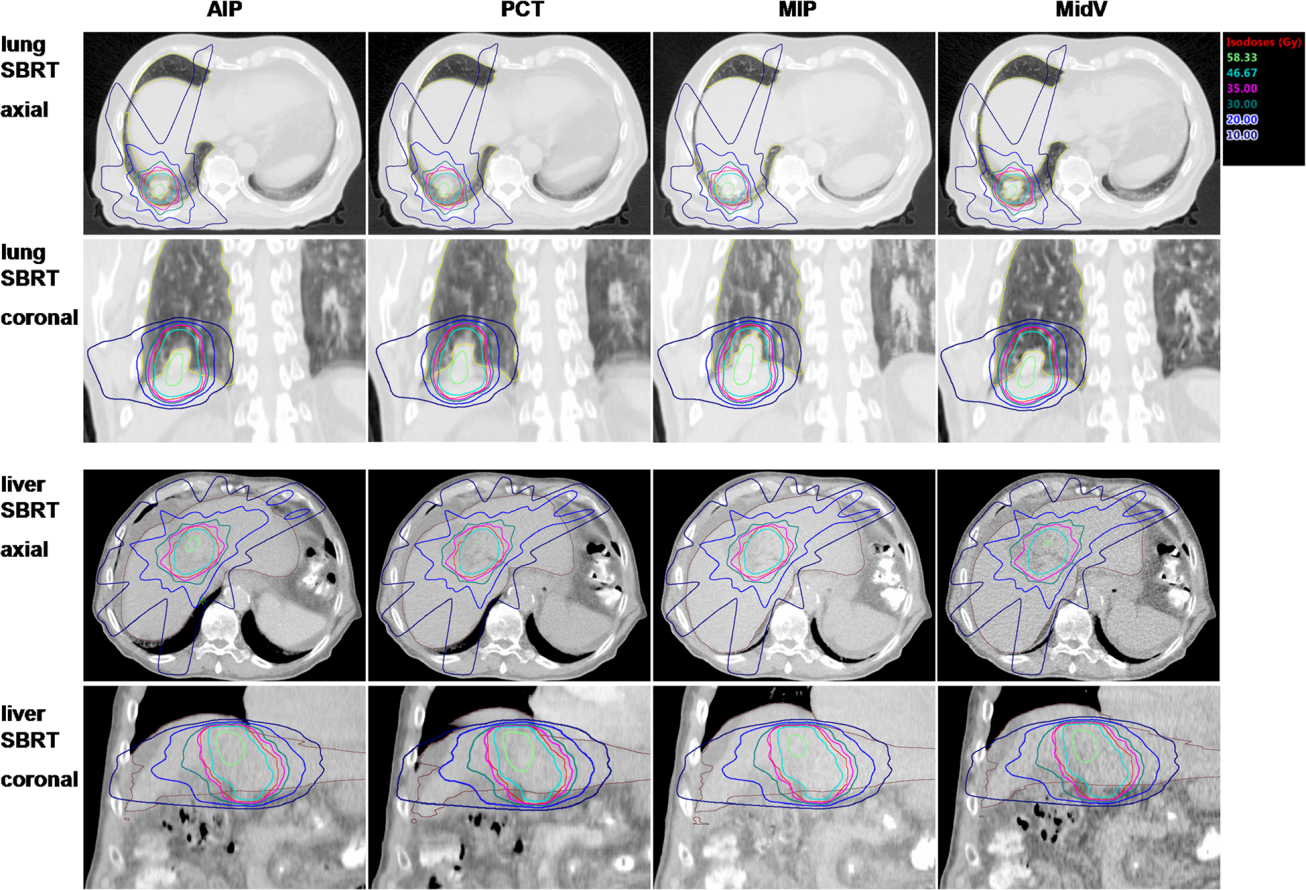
Axial and coronal slices with dose distributions for a lung and a liver SBRT patient. The size of lung and liver appears differently in the CT datasets. PCT: planning CT, AIP: average intensity projection CT, MIP: maximum intensity projection CT, MidV: mid-ventilation CT
